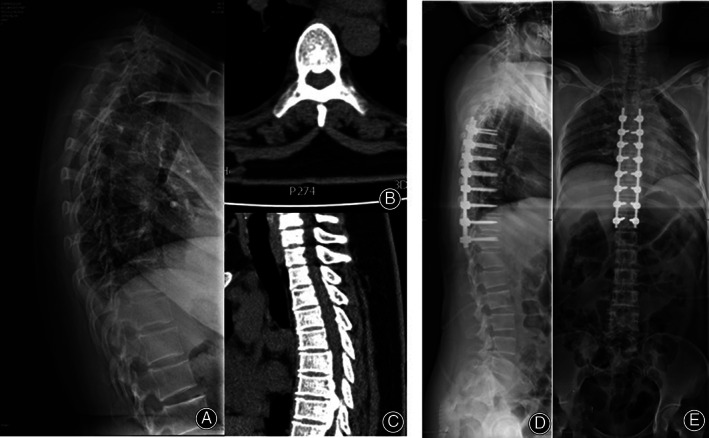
OPLL in a 47‐year‐old female with some neurological symptoms. (A, B, C) Preoperative radiographic images. The blue arrows indicate ossification of the posterior longitudinal ligament in the T_7‐9_ segments. (D, E) Postoperative X‐ray images.
